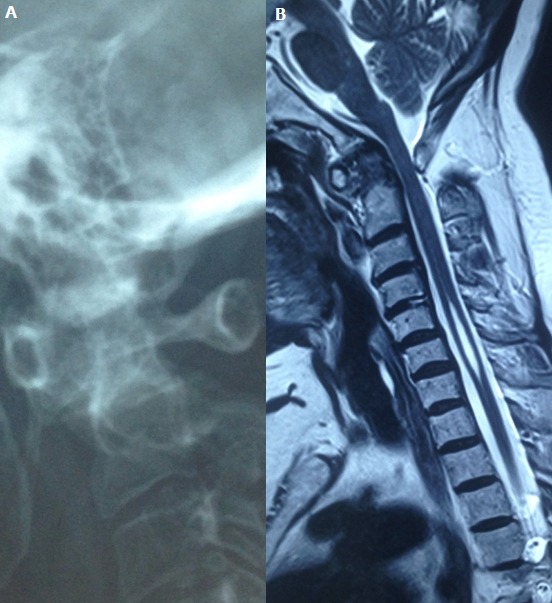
(A) radiograph of a cervical spine showing ananterior atlantoaxial subluxation; (B) MRI showing a pannus around the atlantoaxial joint with a co-existing syringomyelia
